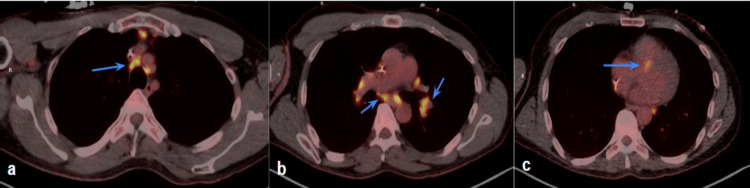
PET-CT chest shows hypermetabolic activity among cardiac and mediastinal structures. (a) mediastinal lymph nodes, (b) perihilar lymph nodes, and (c) interventricular septum. PET-CT: positron emission tomography-computed tomography
